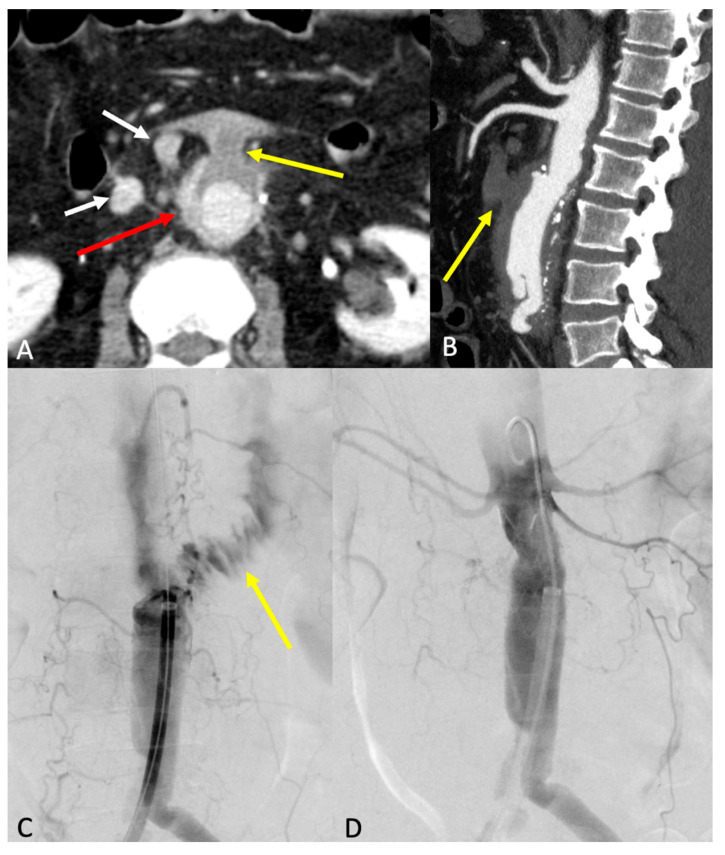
Outcomes of aorto-iliac graft surgery for abdominal aortic aneurysm in CTA (A,B) and angiography (C,D). The images show an infected proximal anastomotic pseudoaneurysm (red arrow in (A)) characterized by a partially thrombosed aorto-duodenal fistula (yellow arrow in (A,B)) and periaortic lymph nodes (white arrows in (A)). The angiographic exam shows the passage of the contrast medium into the duodenum (yellow arrow in (C)) and the subsequent positioning of the endoprosthesis to seal the fistula (D).
